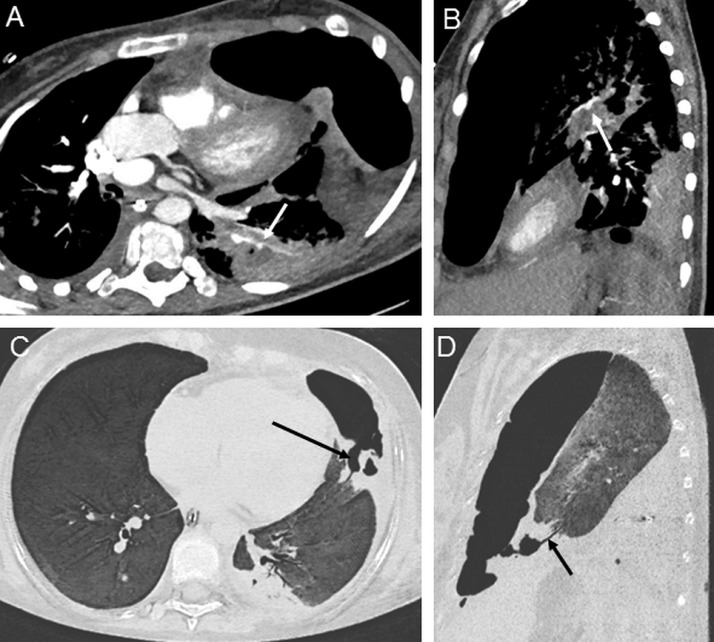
Pulmonary computed tomography angiography (CTA). Thirty days after the baseline chest CT, a second episode of massive hemoptysis occurred that was complicated by heart failure with successful resuscitation. Pulmonary CTA was performed and showed 2 pseudoaneurysms that developed from the subsegmental arteries of the left lower lobe (A) and the anterior segment of the culmen (B) without active bleeding. The invasive aspergillosis lesions were stable, except for the appearance of ground-glass micronodes due to intra-alveolar hemorrhage. A bronchopleural breach (C, D) was visible, which could explain the persistence of pneumothorax despite drainage.
